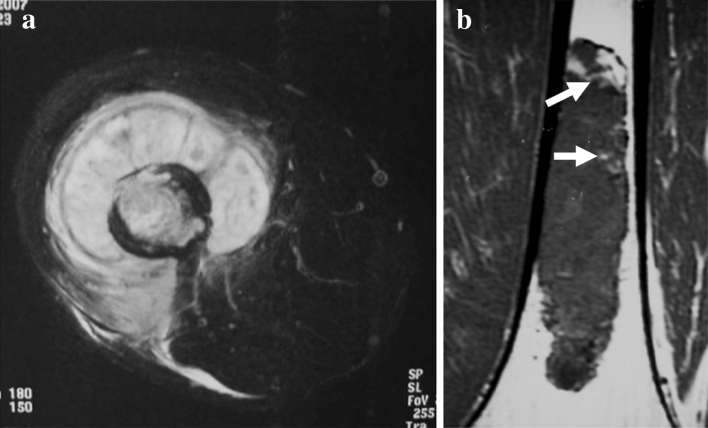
Magnetic resonance imaging findings of chondrosarcoma. a Axial T2-weighted fat-suppressed image showing soft-tissue mass formation. b Coronal T1-weighted image showing entrapped fat (*arrows*), which indicates entrapped areas of pre-existing yellow marrow
